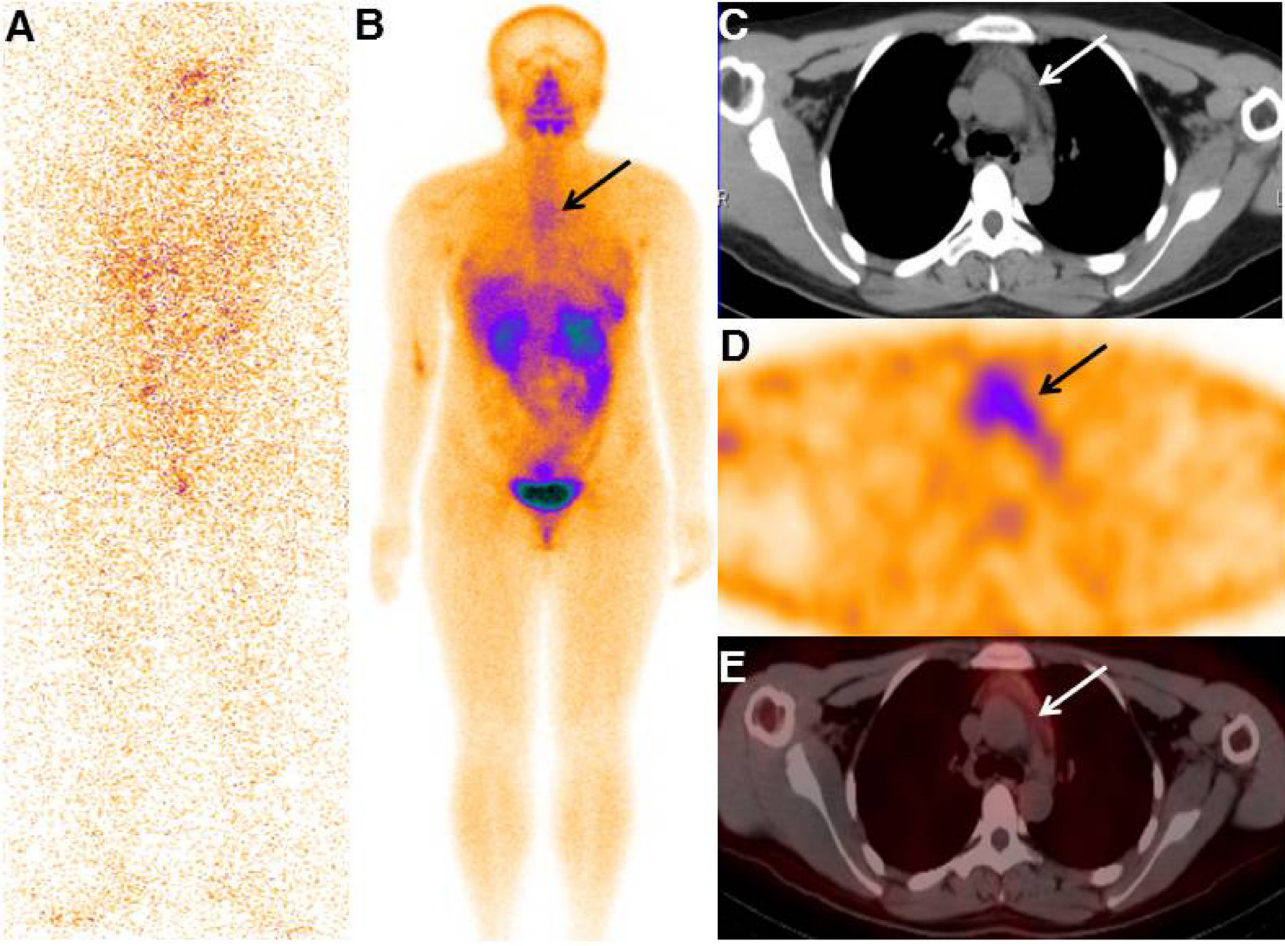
Typical image of the TENIS patient with enlarged thymus that accumulating ^99m^Tc-3PRGD2 The final diagnostic radioiodine scan was negative (A), while whole-body projection SPECT image showed increased ^99m^Tc-3PRGD2 uptake in the mediastinal region (B, arrow). SPECT/CT images showed increased radiopharmaceutical uptake corresponding to the enlarged thymus in the patient (C–E, arrows; from the first to the third row).
